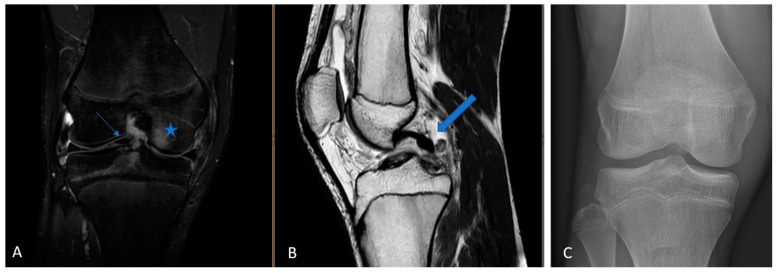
Example of a 3.0 Tesla knee MRI: (A) coronal PD tse fs, (B) sagittal T2 tse, and (C) conventional X-ray in AP view of 14-year-old male patient with open physis and acute ACL rupture. PD tse fs (proton density-weighted turbo spin echo sequence with fat saturation), T2 tse (T2-weighted turbo spin echo sequence), ap (anterior–posterior); large arrow is ACL rupture; star is bone marrow edema.
